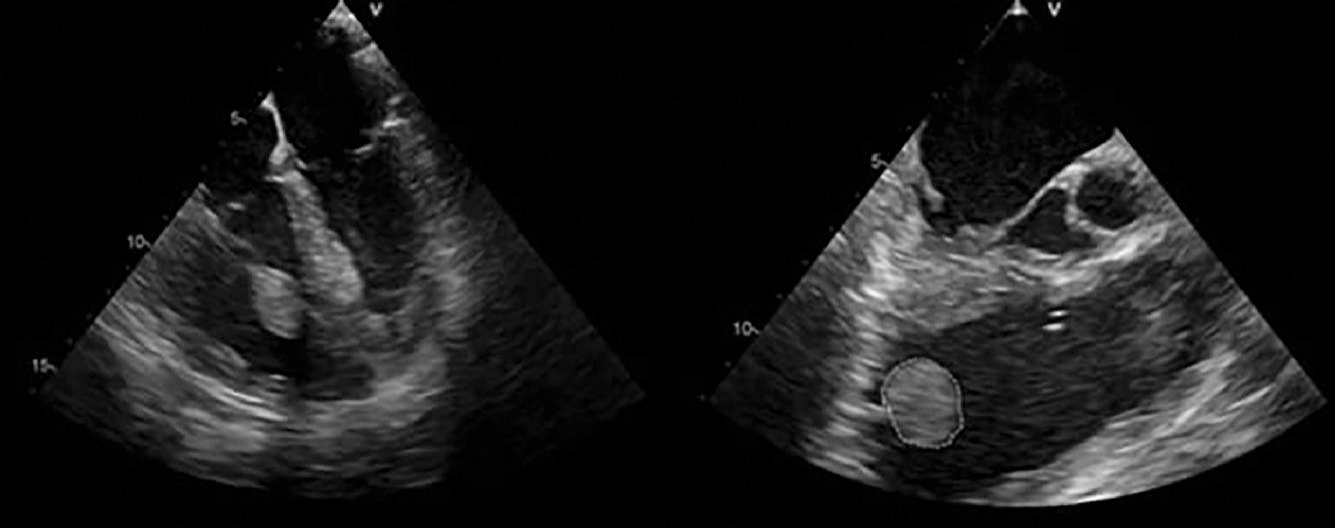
Transoesophageal cardiac echocolordoppler showing thrombus on pacing lead in the right ventricle (area 2.9 cm^2^).
